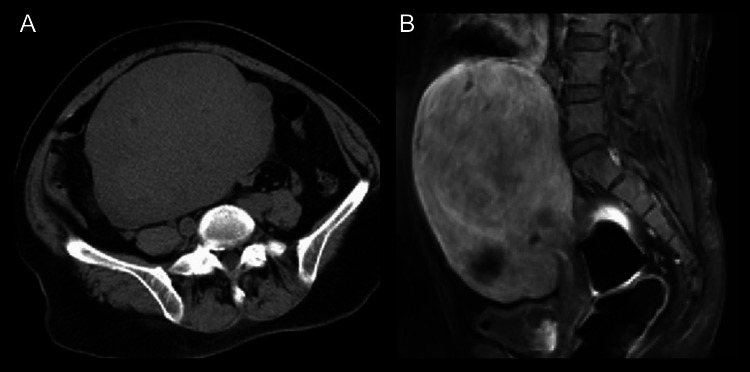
Pelvic tumor Internally uniform pelvic tumor with smooth edges was observed during axial CT (A). T2-weighted magnetic resonance imaging suggested no malignancy (B).
